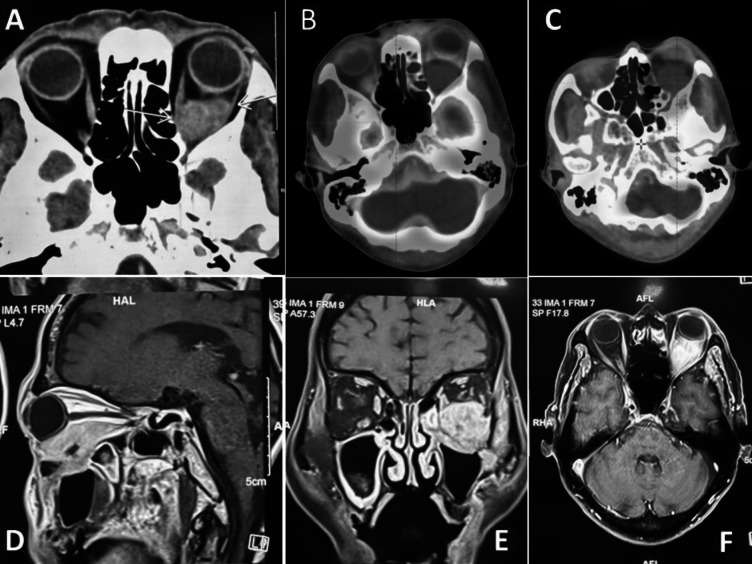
Pre-treatment images. a Axial CT; b, c axial PET-CT; d CEMRI sagittal, e CEMRI coronal, and f CEMRI axial showing an irregular mass lesion in the left orbit in retroorbital space predominately in the inferior part
